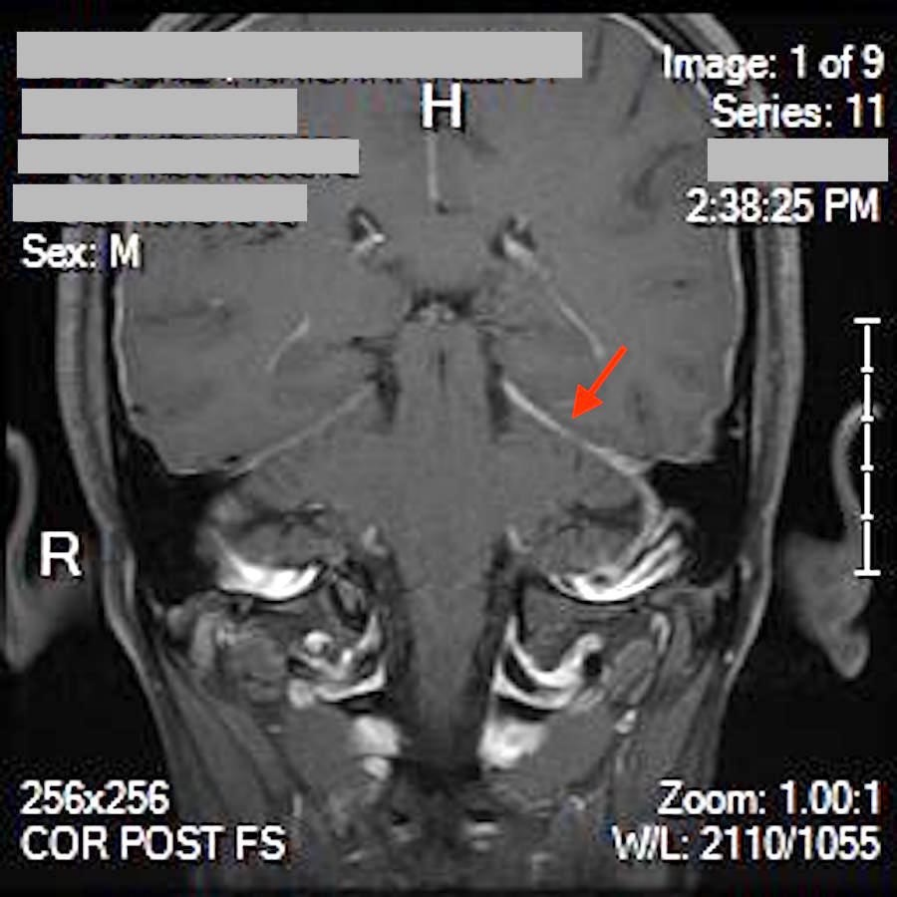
MRI brain without contrast, showing asymmetric tentorial enhancement (red arrow) MRI: magnetic resonance imaging
